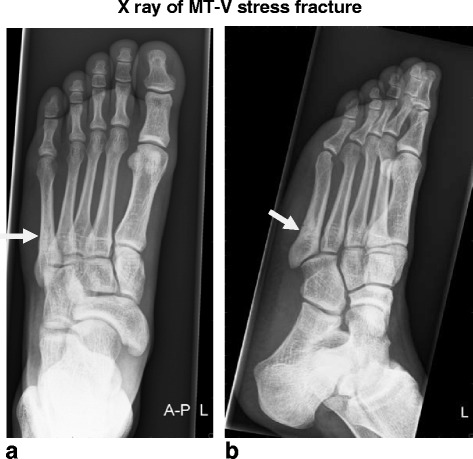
X-ray of MT-V stress fracture. a. AP view b. Oblique view. Stress fracture of the MT-V, located in the metaphyseal-diaphyseal junction also called zone 2; or just distally from the intrametatarsal joint (MT-IV/MT-V) in the proximal diaphysis also called zone 3 [45], with sclerosis seen on both fracture sidesSkeletally mature
